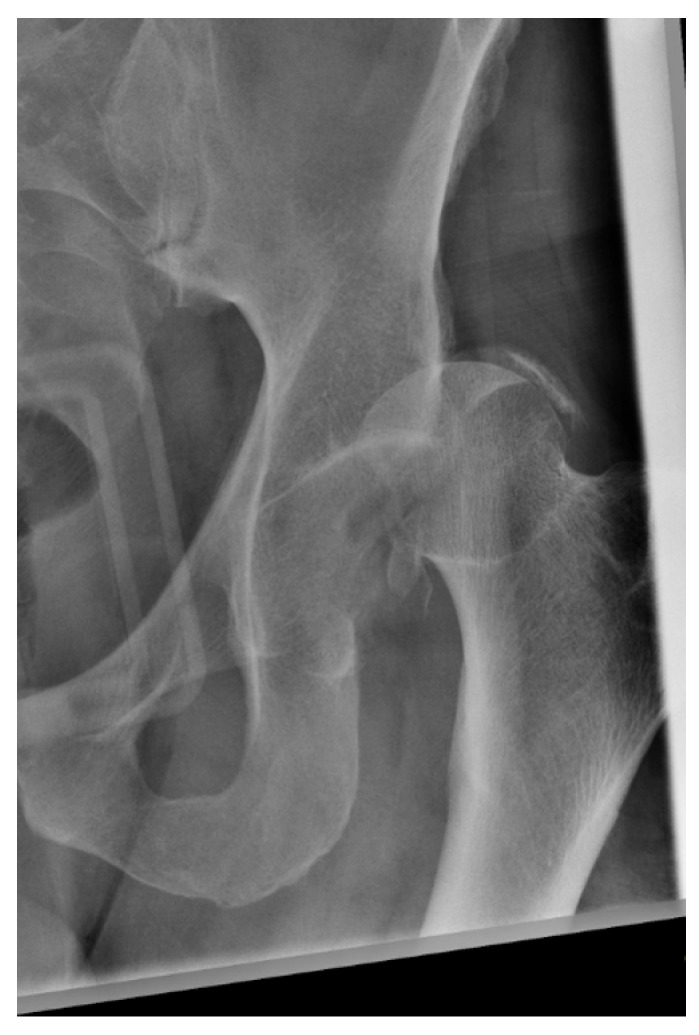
AP X-ray of a pelvis showing a Pipkin 4 fracture involving the acetabulum with a hip dislocation in the shock room.
